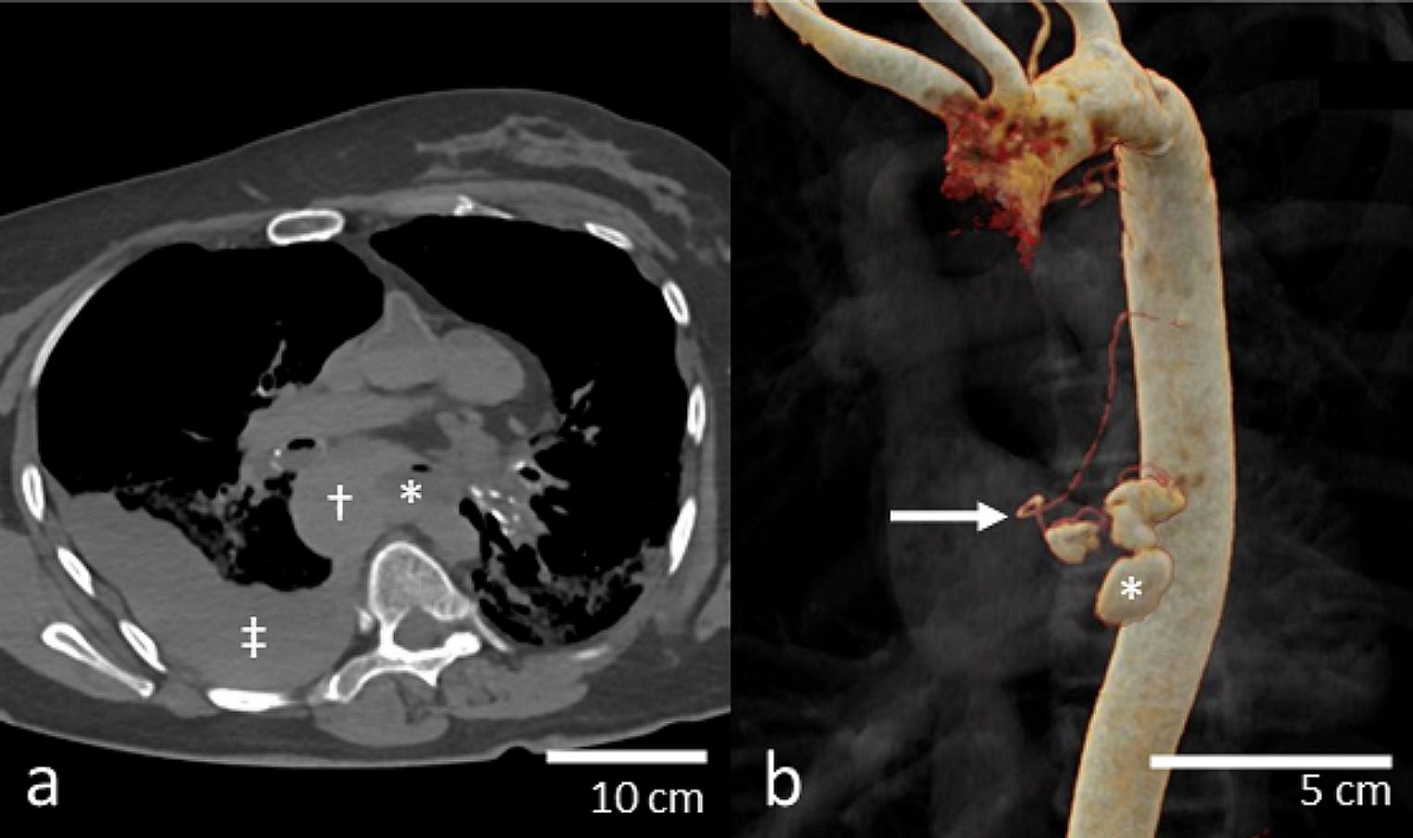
Post-mortem CT and post-mortem CT angiography findings. (a) Transverse reconstruction of the thoracic region of non-contrast post-mortem CT, demonstrating the esophagus (*) and paraesophageal hematoma (†), which is continuous with a substantial right-sided hemothorax (‡). (b) 3D-reconstruction of the arterial phase of the post-mortem CT angiography, demonstrating an esophageal branch of the descending aorta (arrow) with contrast leak into the mediastinal hematoma (*)
